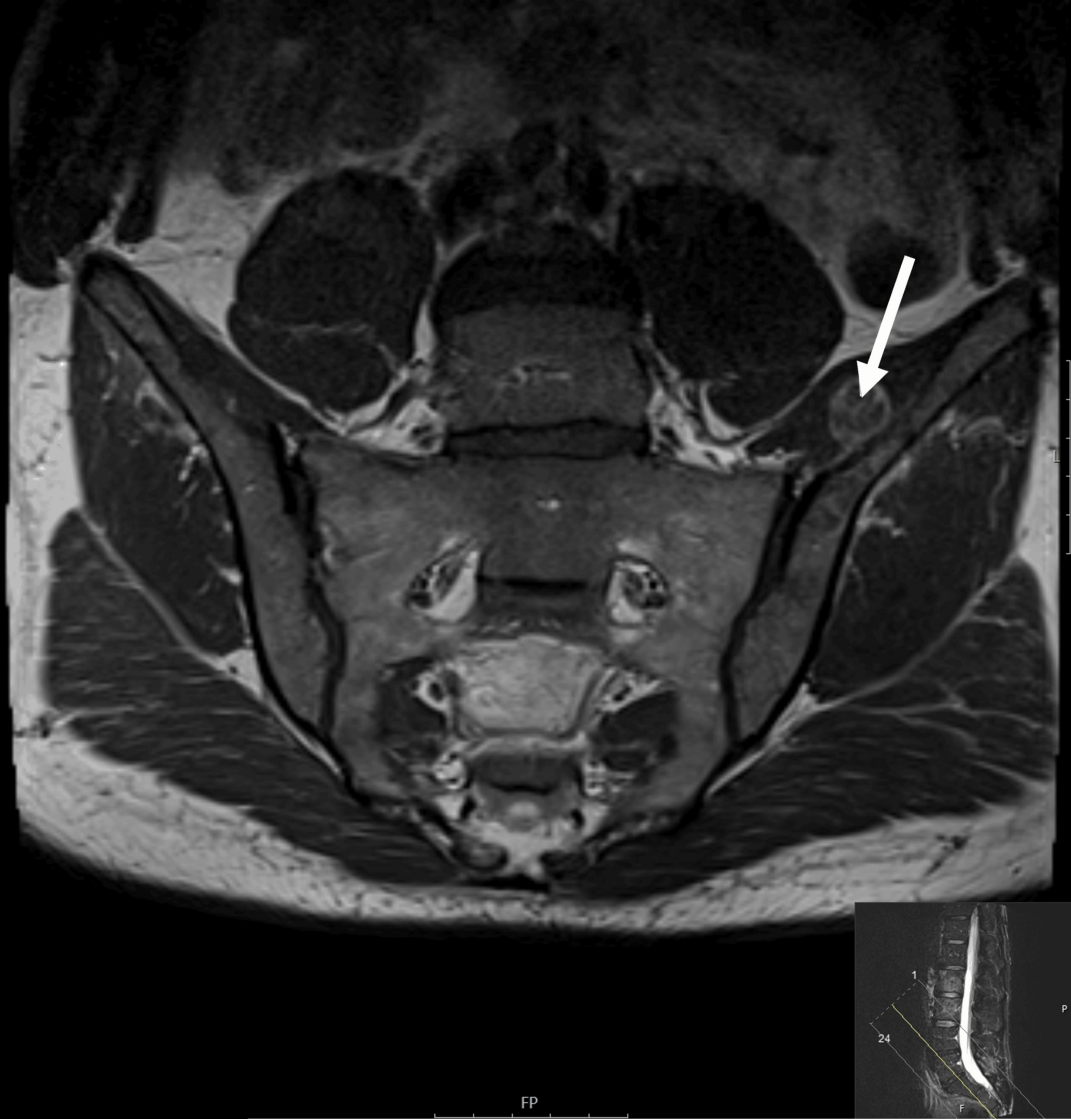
Magnetic resonance imaging T1-weighted imaging (MRI T1W1) sequence: axial plane Neoplastic invasion of the sacrum and of the iliac bone, with an extension to the iliac muscle (arrow)
